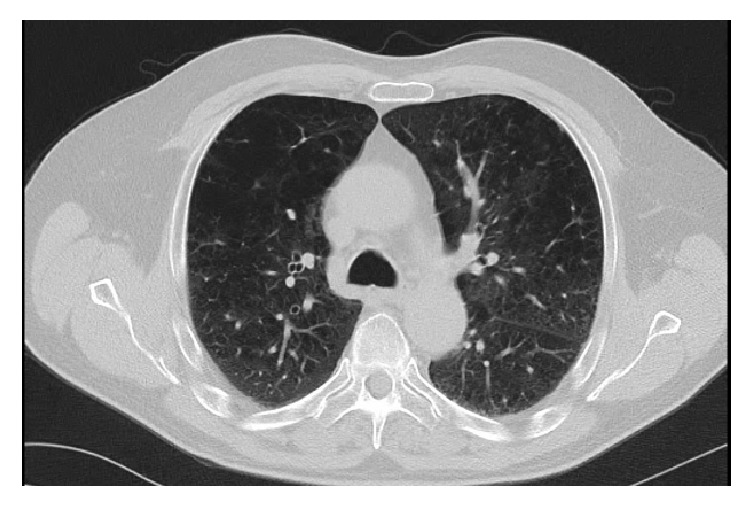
Repeat Chest CT, after one week of the initial CT, showing almost complete resolution of previous infiltrates, disappearance of mediastinal lymphadenopathy, and resolution of bilateral pleural effusions.
